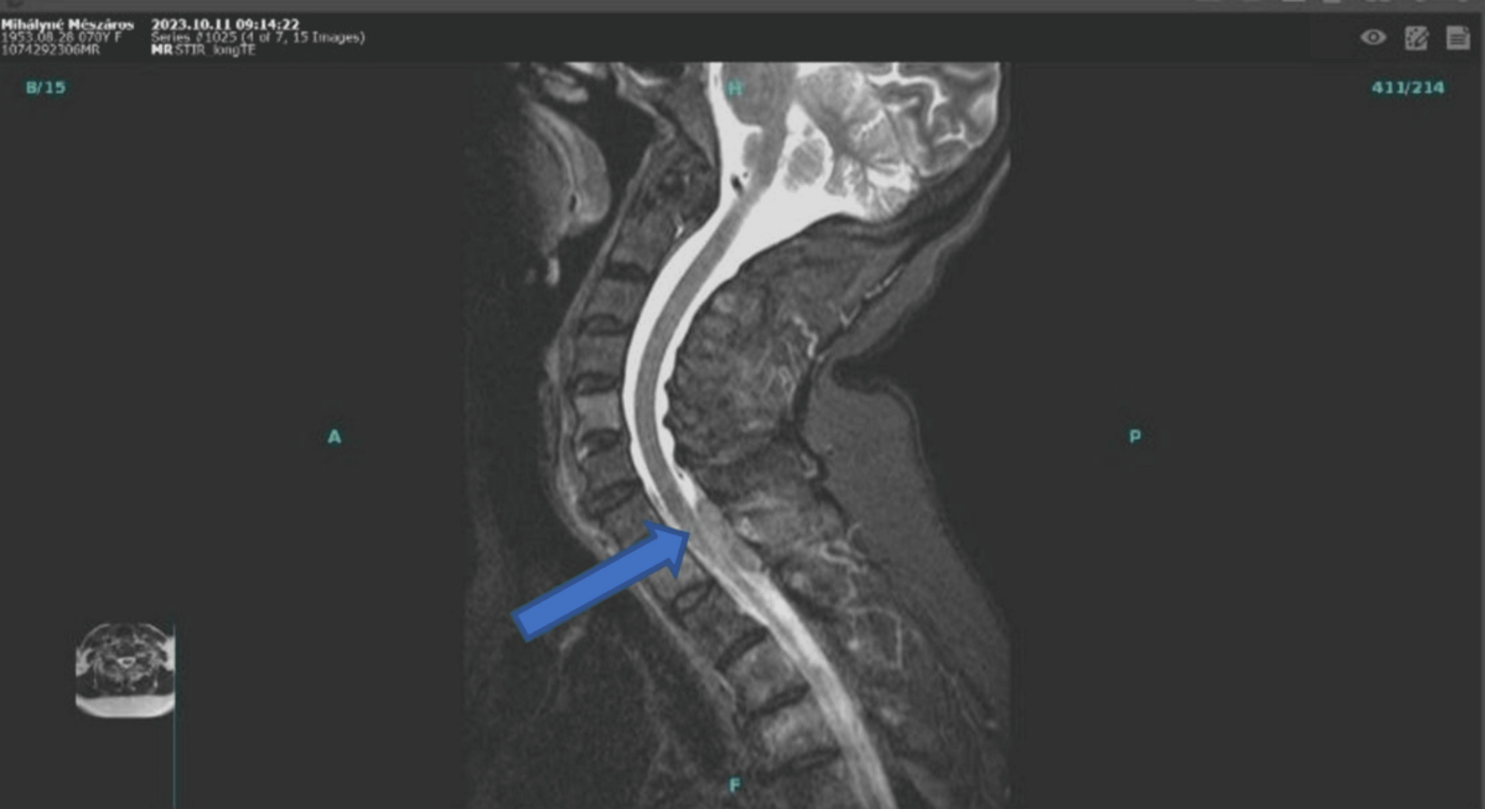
MRI showing intra- and extramedullary mass extending from cervical vertebrae (C1-C7) and the first thoracic vertebra (Th1) (arrow)
